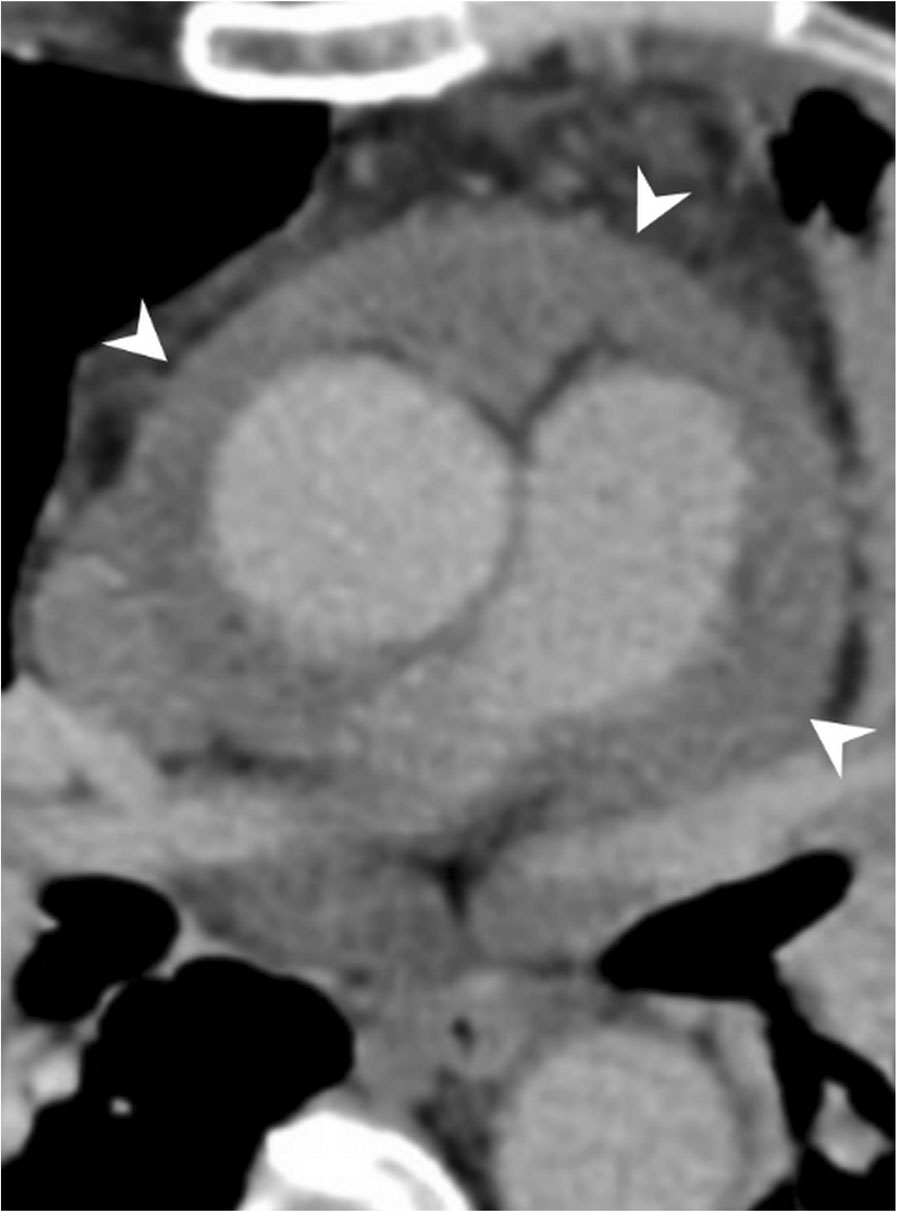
A 52-year-old woman with breast cancer receiving radiotherapy for treatment. Axial contrast-enhanced CT image reveals pericardial effusion with increased density compatible with hemopericardium (arrowheads)
